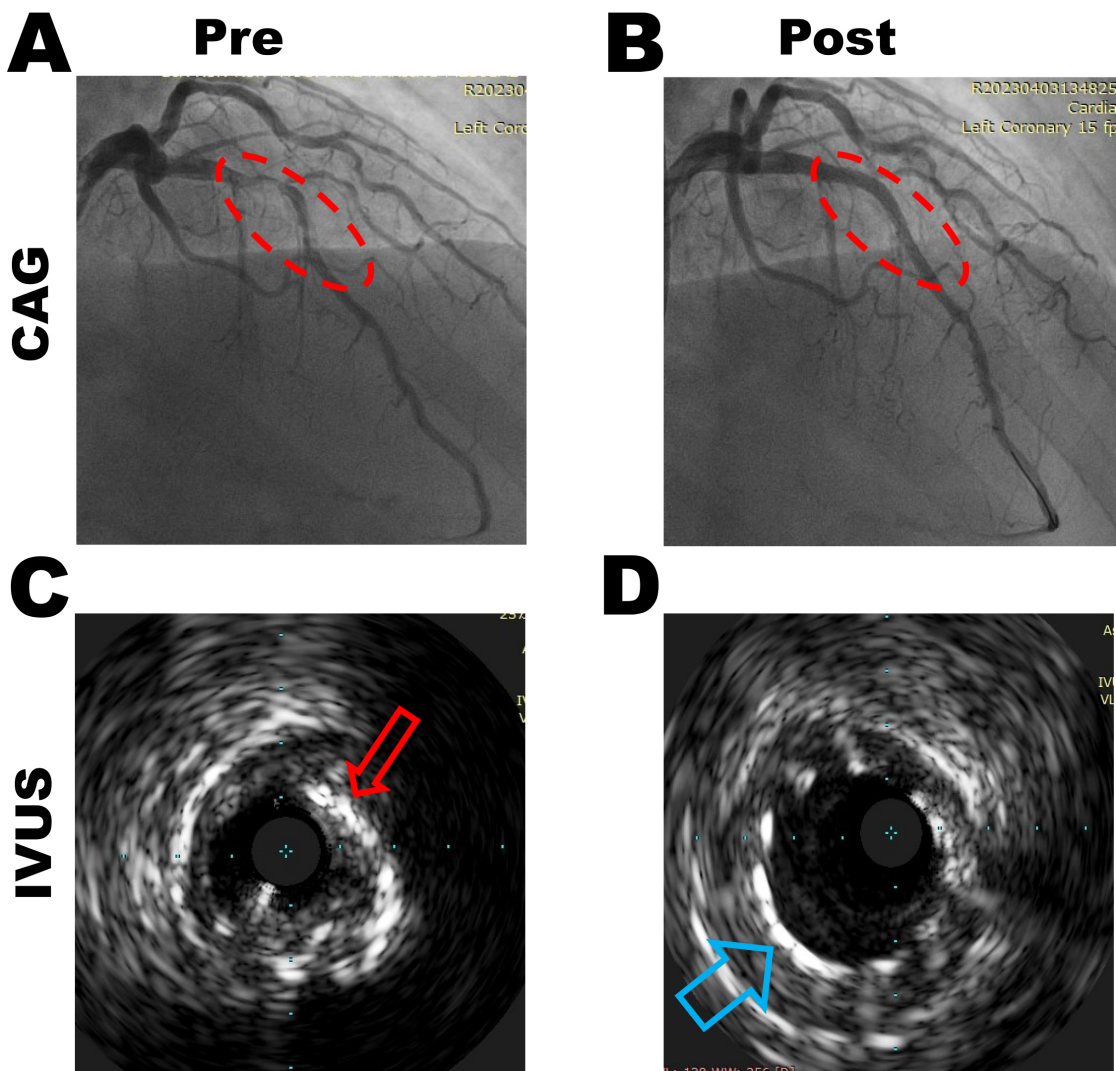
Intravascular ultrasound guiding percutaneous coronary
